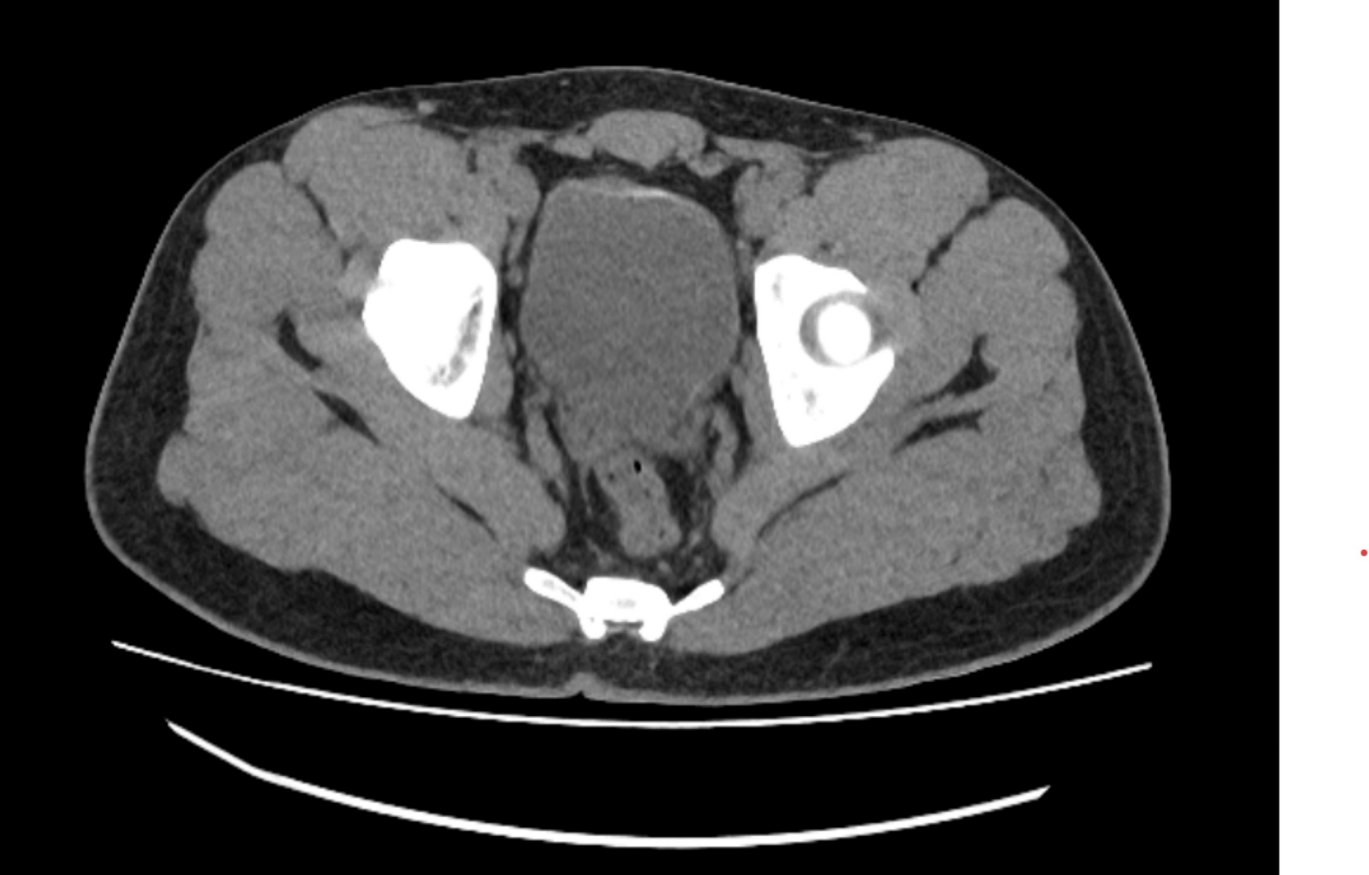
Axial non-contrast CT scan showing circumferential linear calcifications of the bladder wall
